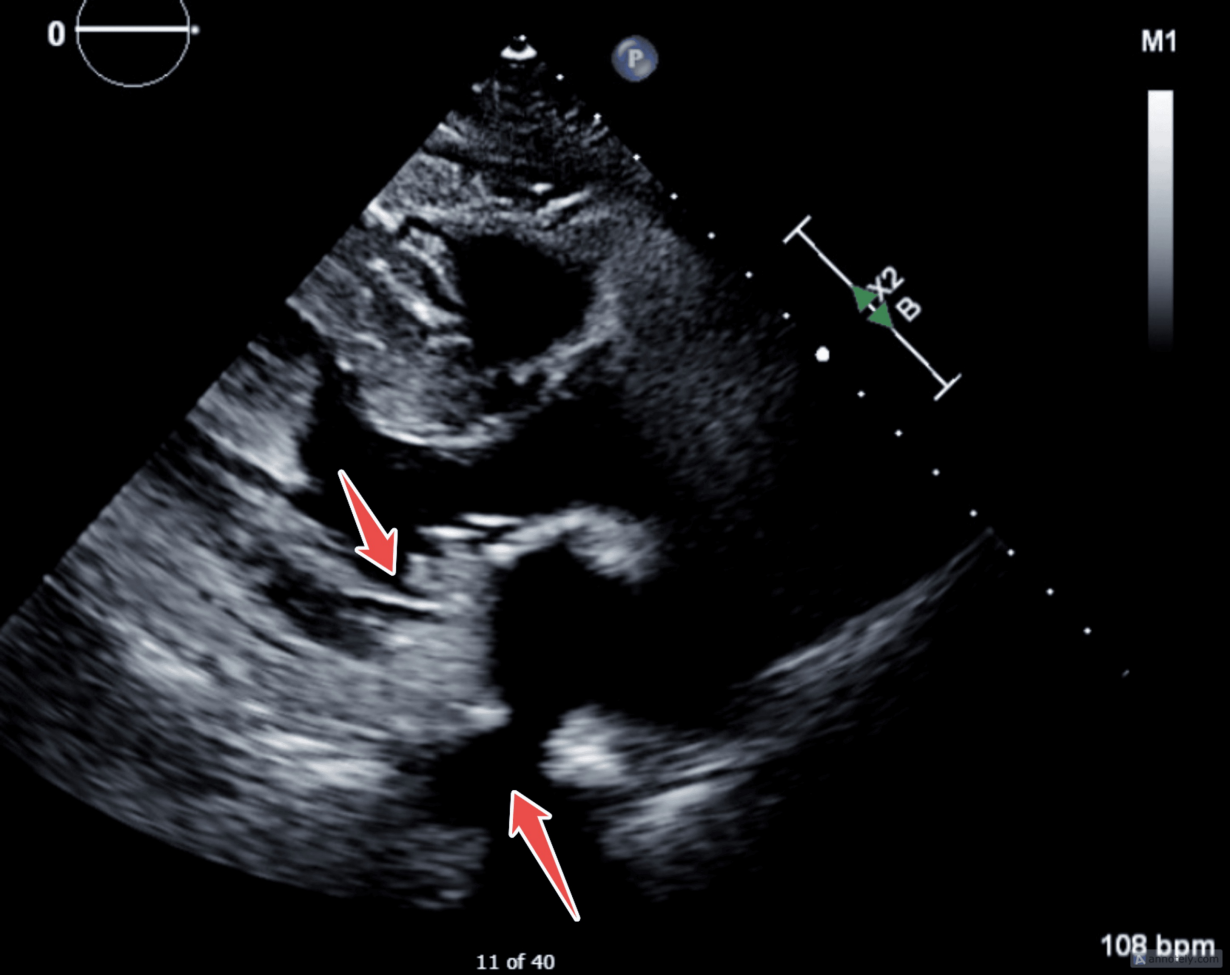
TTE in PLAX view post mechanical mitral valve replacement highlighted in arrows. TTE: transthoracic echocardiogram, PLAX: parasternal long axis
